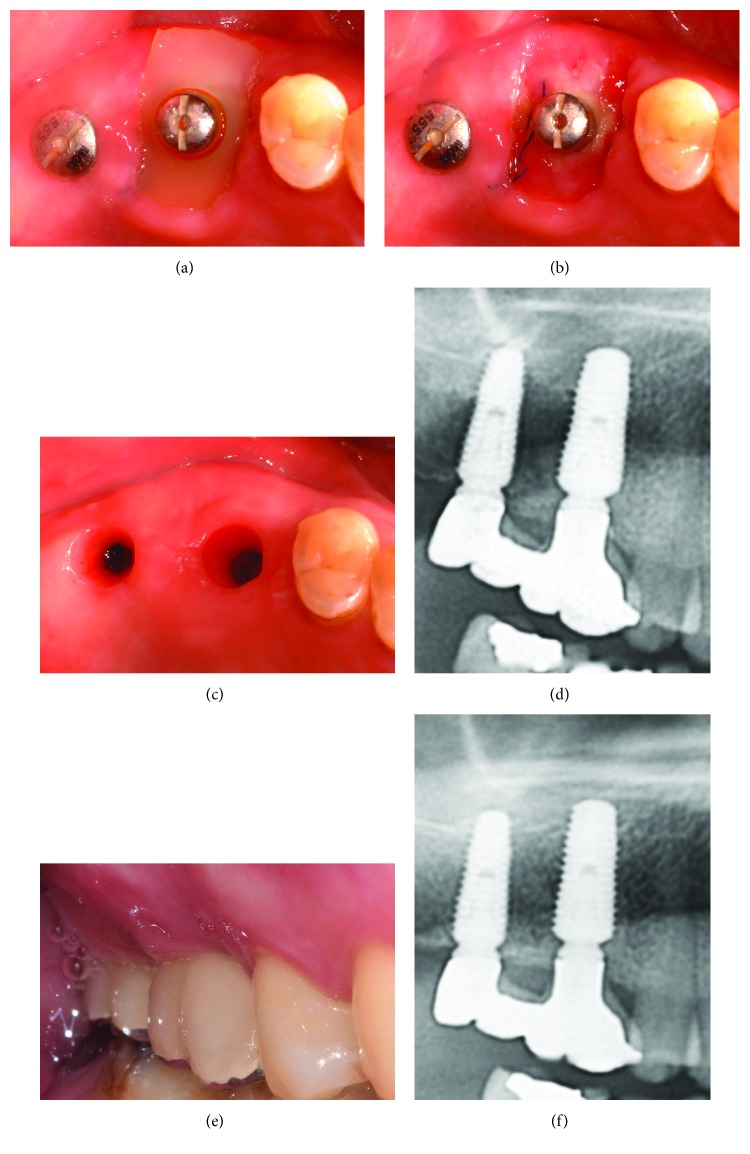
(a) Clean silicone cap at 2 weeks postoperatively. (b) Healing condition of the extraction socket at 2 weeks postoperatively. (c) Clinical condition before the application of the final prosthesis at 8 months after surgery. (d) Radiograph taken after the application of the final prosthesis at 8 months after surgery. (e) Buccal view at 2 years after surgery. (f) Radiographic view at 2 years after surgery.
